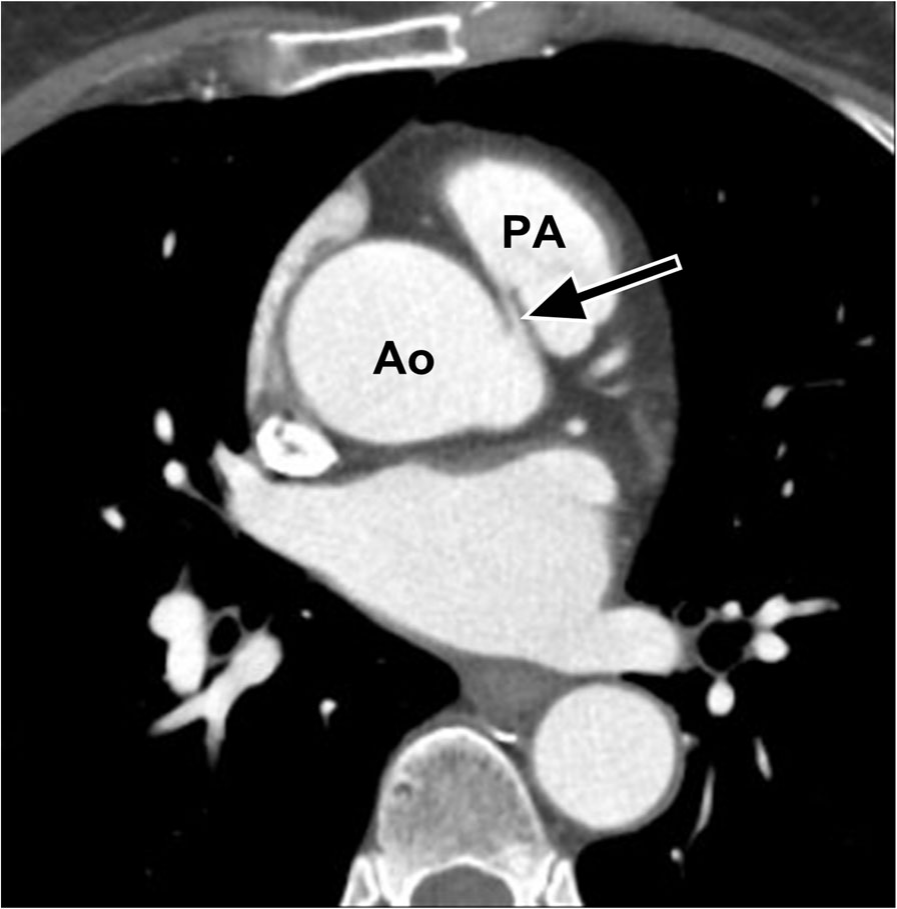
Axial contrast enhanced CT demonstrates the RCA (black arrow) arising from the left coronary cusp in a 43-year-old female being evaluated for hoarseness and vocal cord paralysis. *Ao* aorta, *PA* pulmonary artery
